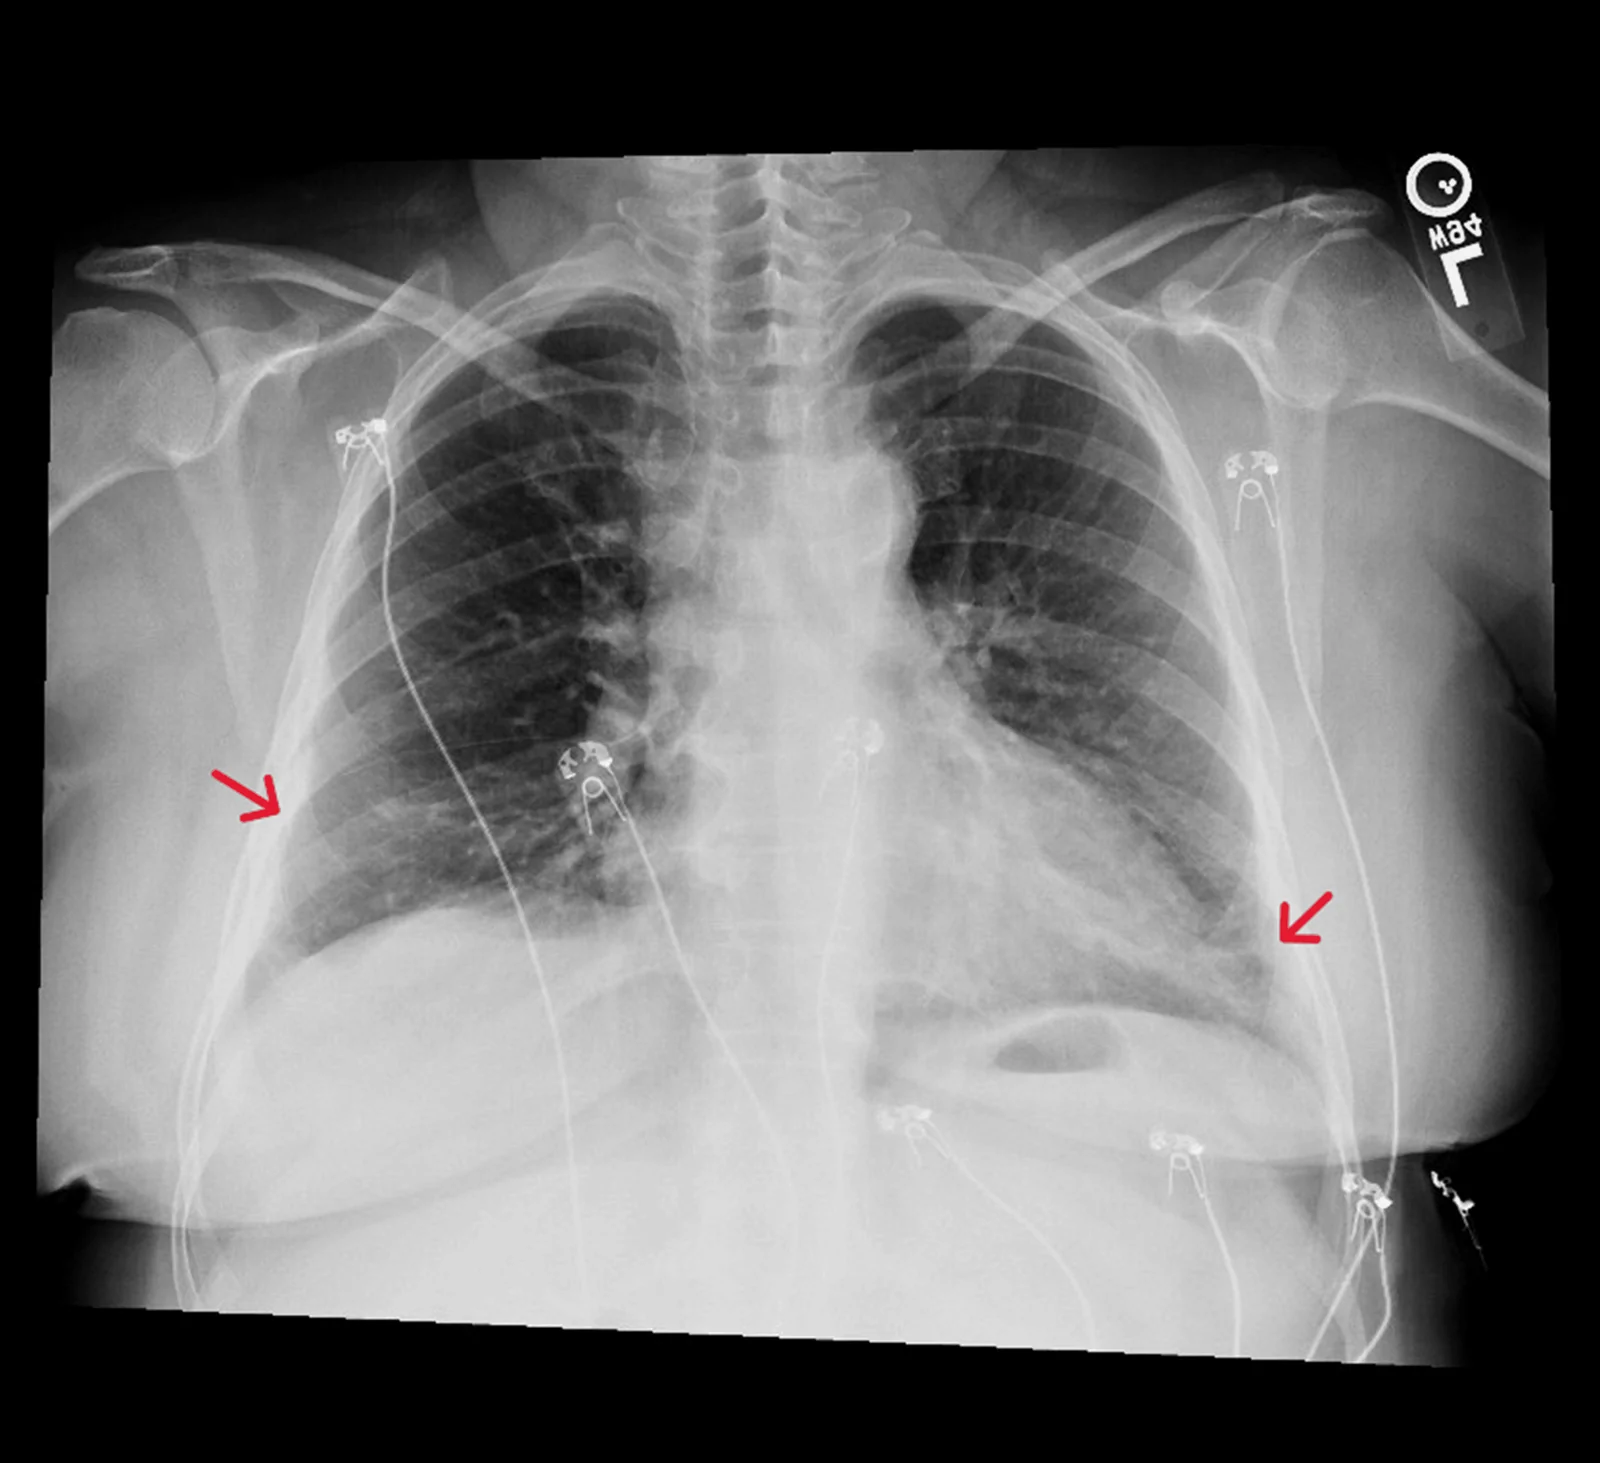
Initial chest radiograph obtained during the patient's first emergency department presentation demonstrating patchy bibasilar opacities. Anteroposterior chest radiograph. Red arrows indicate patchy bibasilar airspace opacities. These nonspecific findings were initially interpreted as concerning for atelectatic change versus early pneumonia.
